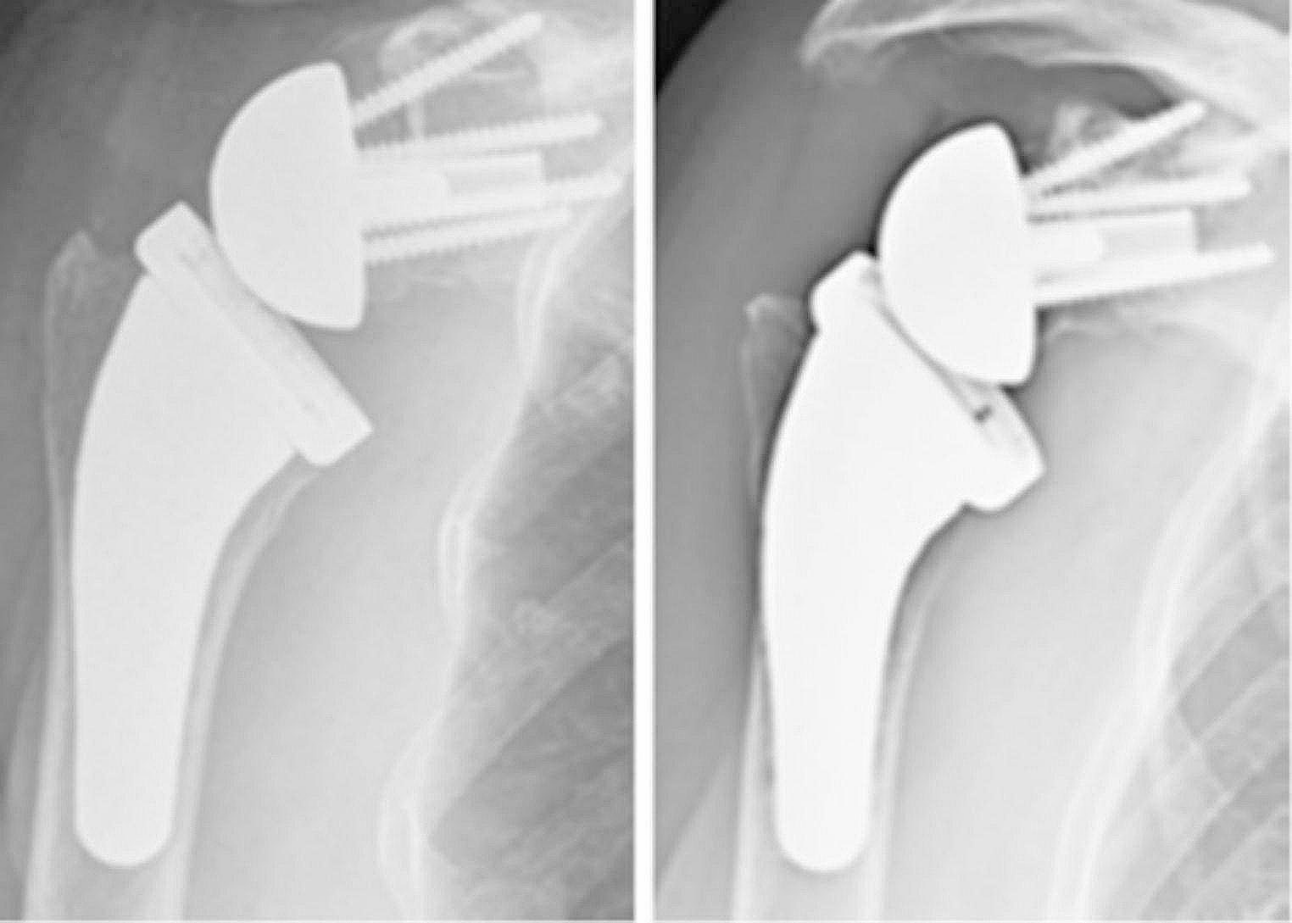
A patient with cortical bone narrowing and osteopenia at the lateral proximal zone and medial proximal zone. Left - immediate postoperative radiograph. Right - at 37 months follow-up
